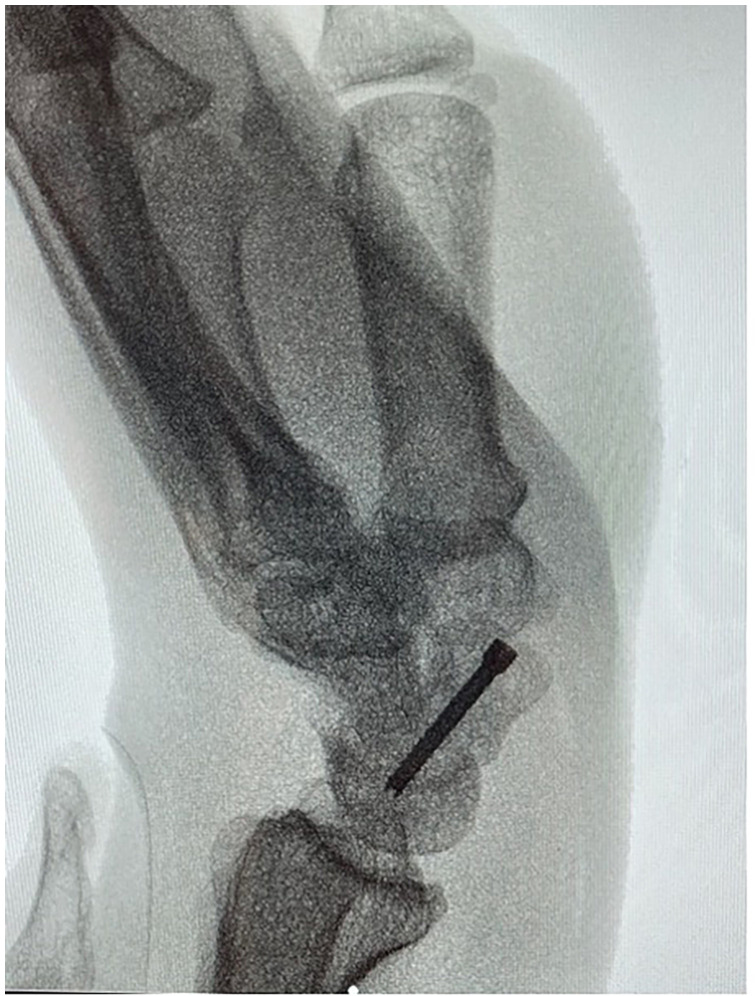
Lateral X-ray post screw placement.
